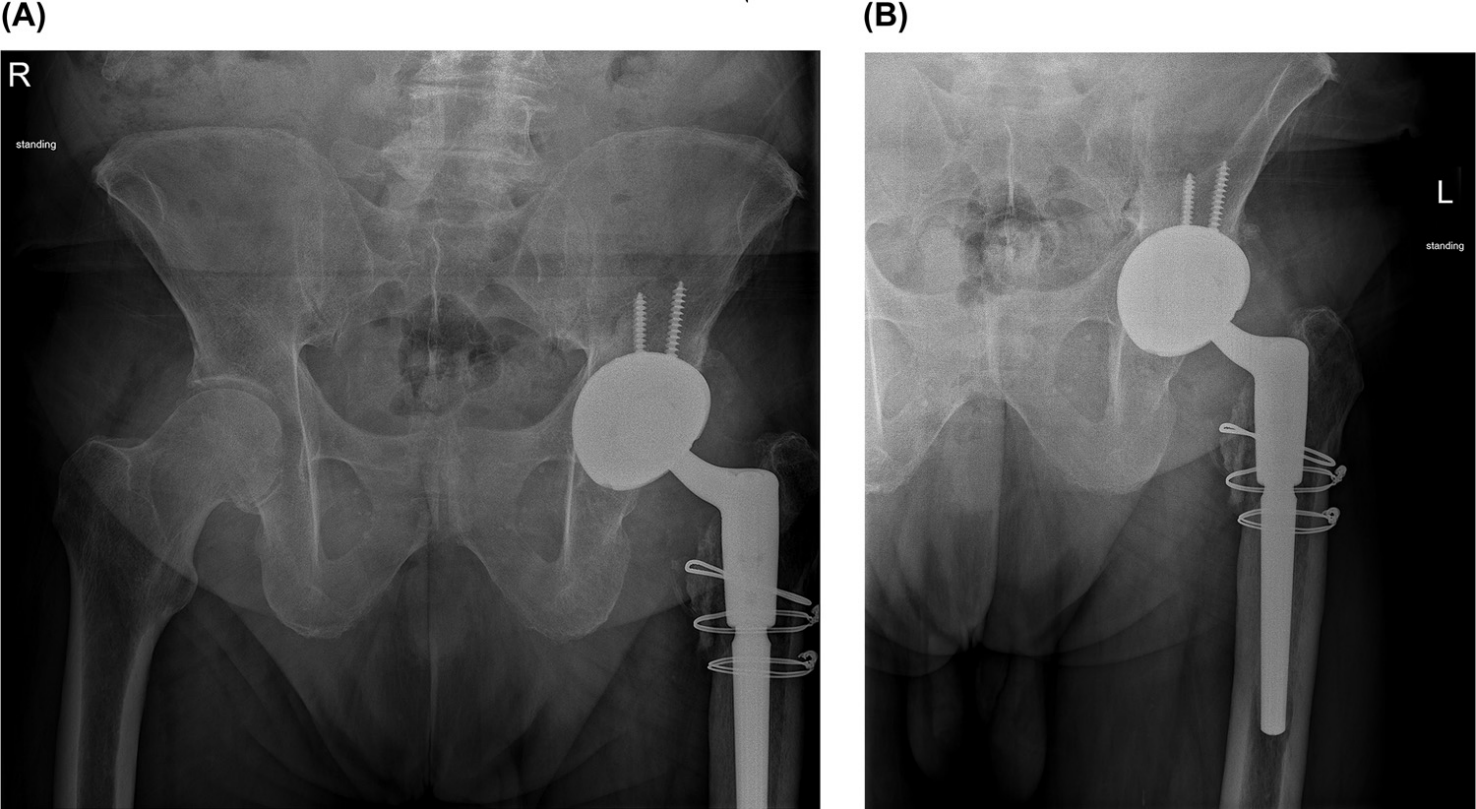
Radiographs of the (A) pelvis and (B) left hip obtained 6 months after revision surgery demonstrate revised left total hip arthroplasty with well-seated components. The left lesser trochanter fracture sustained during surgery and fixed with cerclage cables appears to be healing.
